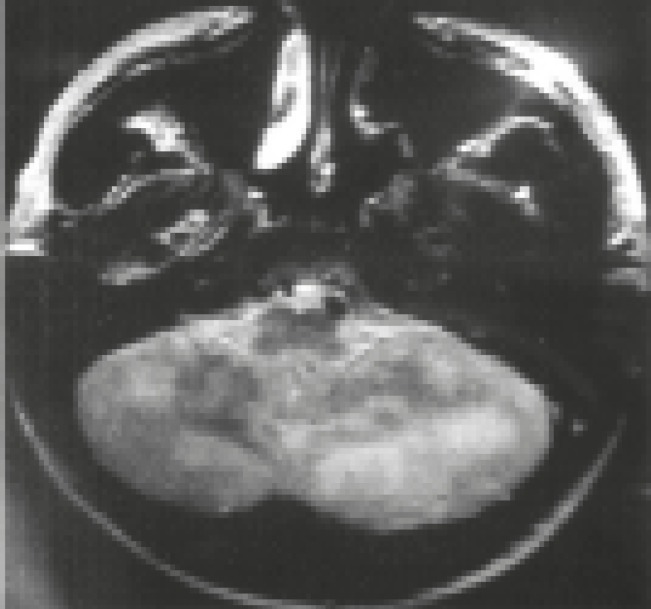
T2-weighted MRI of the brain demonstrating foci of high signal in the cerebellum
